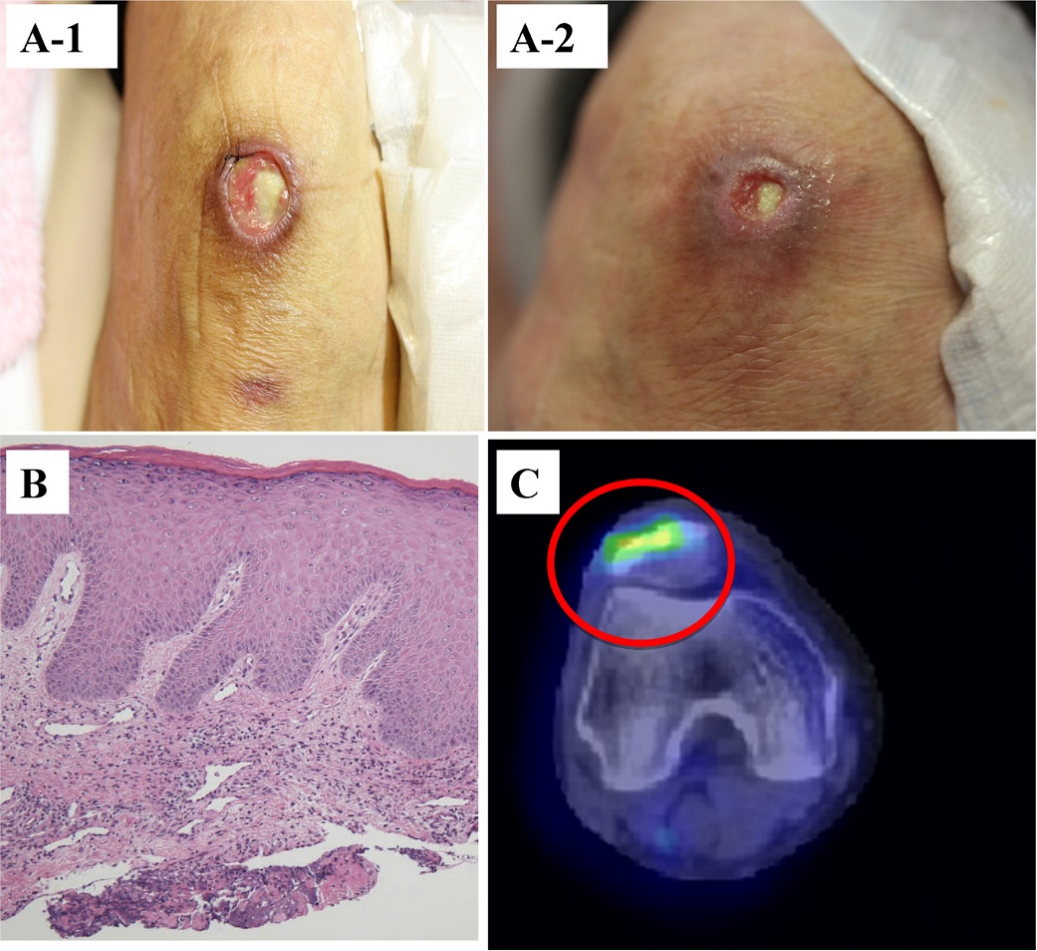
Cutaneous ulceration before and after steroid therapy; pathology and PET/CT findings of the cutaneous ulceration. (A-1) The right knee cutaneous ulcer measured 4 cm before steroid therapy. (A-2) One month after the treatment, it measured < 1 cm and showed granulation tissue growth. (B) A biopsy of the edge of the ulcer revealed a mild lymphocytic infiltrate in the superficial dermis; however, no obvious findings of vasculitis were observed. (C) FDG PET/CT demonstrated increased tracer uptake in the ulcer, suggesting inflammation.
